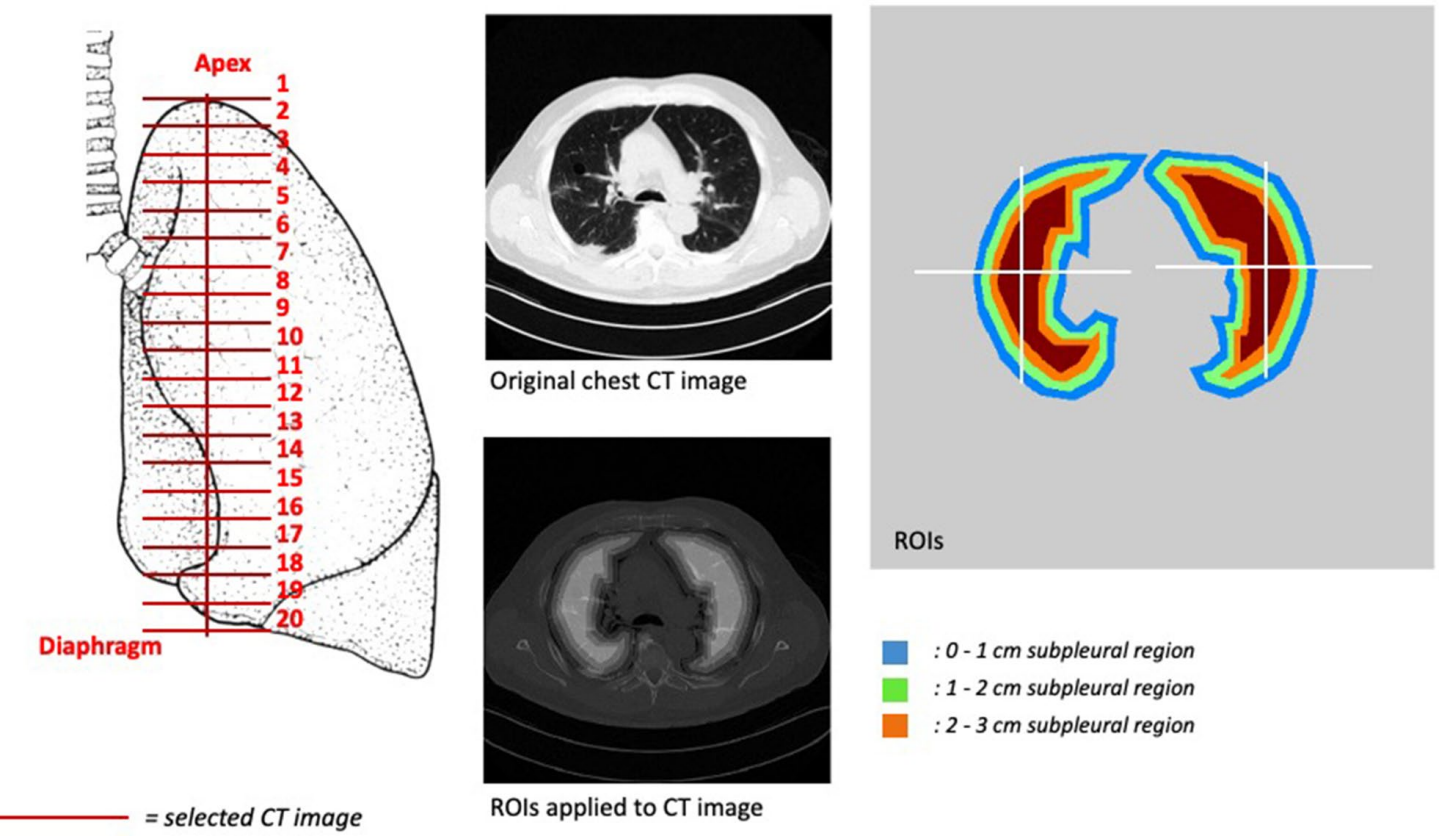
CT image analysis. Left panel. Coronal representation of lungs. Twenty CT images were selected along the cranial-caudal axis. Central panel. Upper part: representative image of an original CT image from a patients included in the study. Lower part: region of interest (ROI) applied to CT image. Right panel: representative image of three ROIs (areas concentric to the visceral pleura: between 0 and 1 cm in blue; 1 and 2 cm in green; and 2 and 3 cm in orange) and quadrants: internal dependent, external dependent, internal non-dependent + external non-dependent). The identification of quadrants was based on the detection of the centroid (or geometrical center) univocally characterizing each lung for each analyzed CT image
